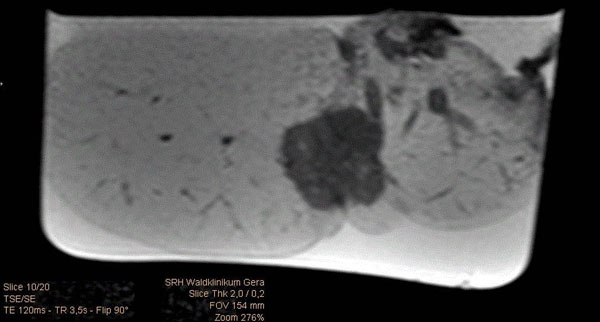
Flooded lung lobe containing NSCLC in T2w MR image
